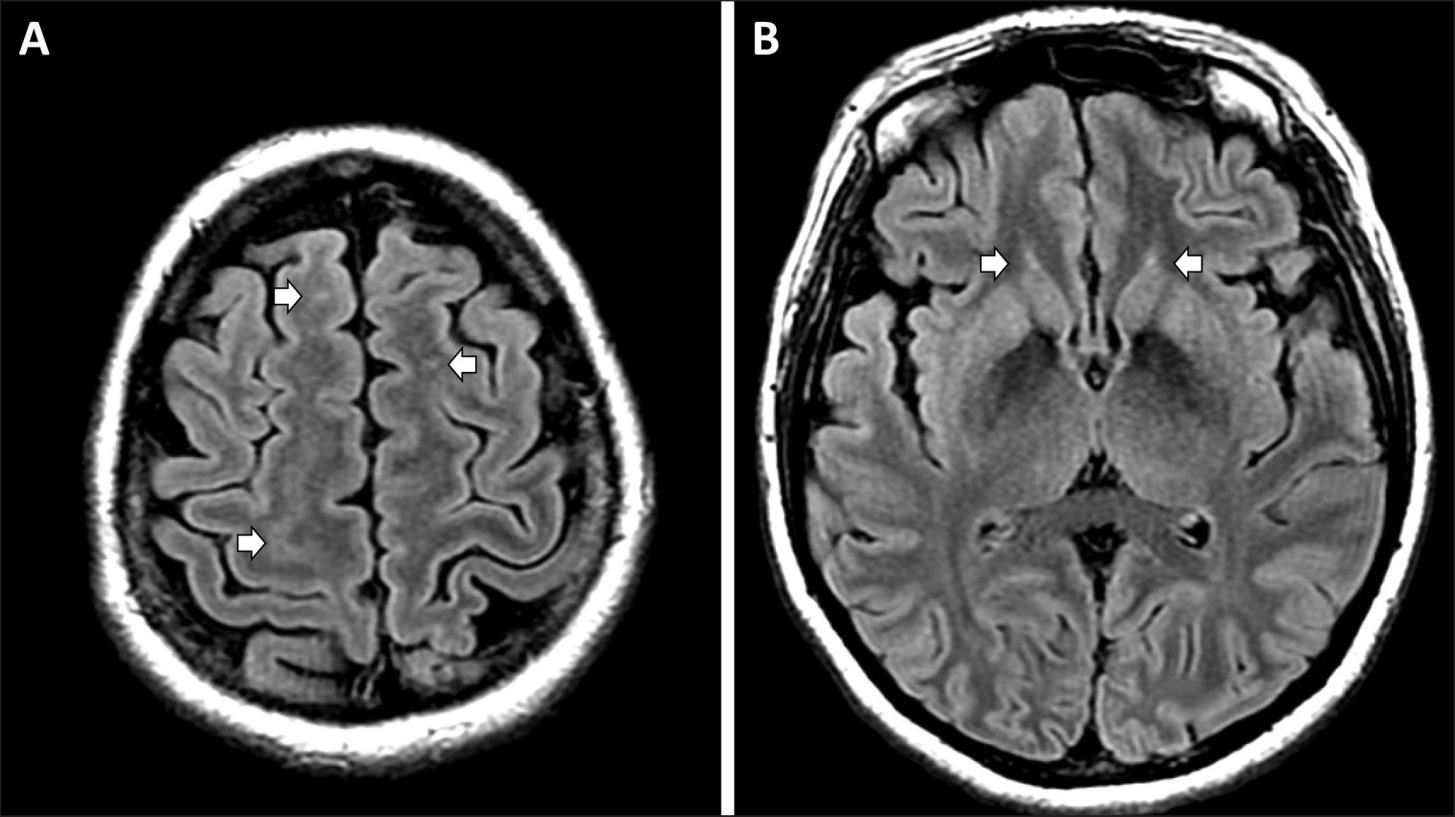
Preoperative MRI. A. Axial FLAIR-weighted preoperative MRI image showing diffuse cortical FLAIR signal hyperintensities as well as areas of FLAIR signal changes in the corona radiata (arrows). B. Axial FLAIR-weighted MRI image showing subtle FLAIR hyperintensities in the head of the bilateral caudate nuclei (arrows).
